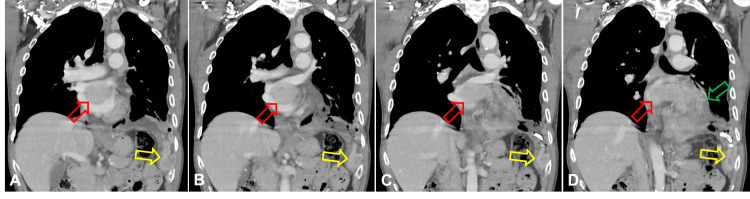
CT scan of chest at initial presentation (May 11, 2020). A-D: Subsequent coronal views. Red arrow: Tumor invasion into the left atrium from left inferior pulmonary veins. Yellow arrow: Pleural metastasis. Green arrow: Metastatic tumor mass occupying left lower lobe and pleura.
